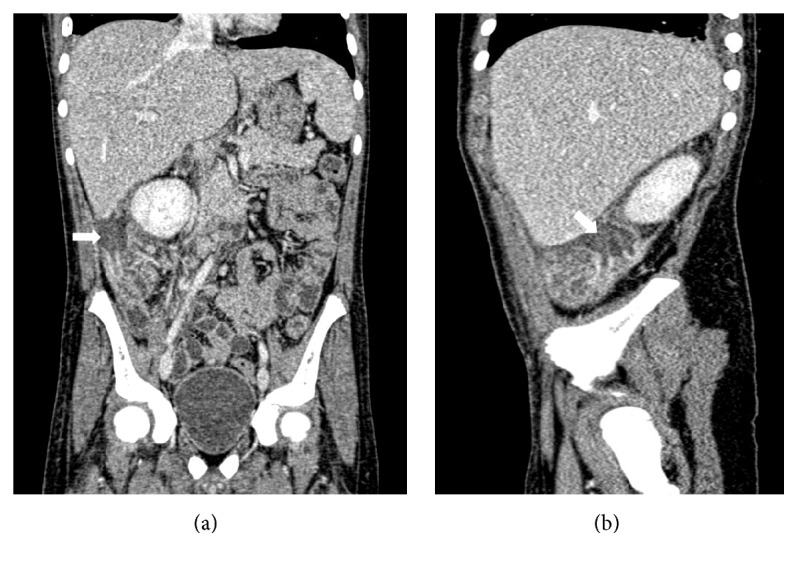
Contrast enhanced CT with coronal (a) and sagittal (b) images showing ruptured (arrow) appendix at the distal body with localized periappendicular collection.
